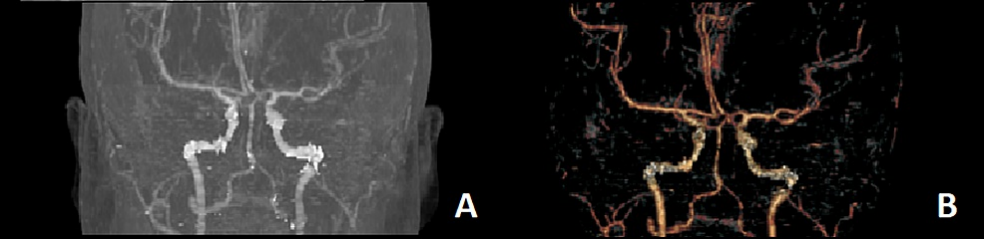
CT angiography showing no vascular abnormality in the posterior circulation.
